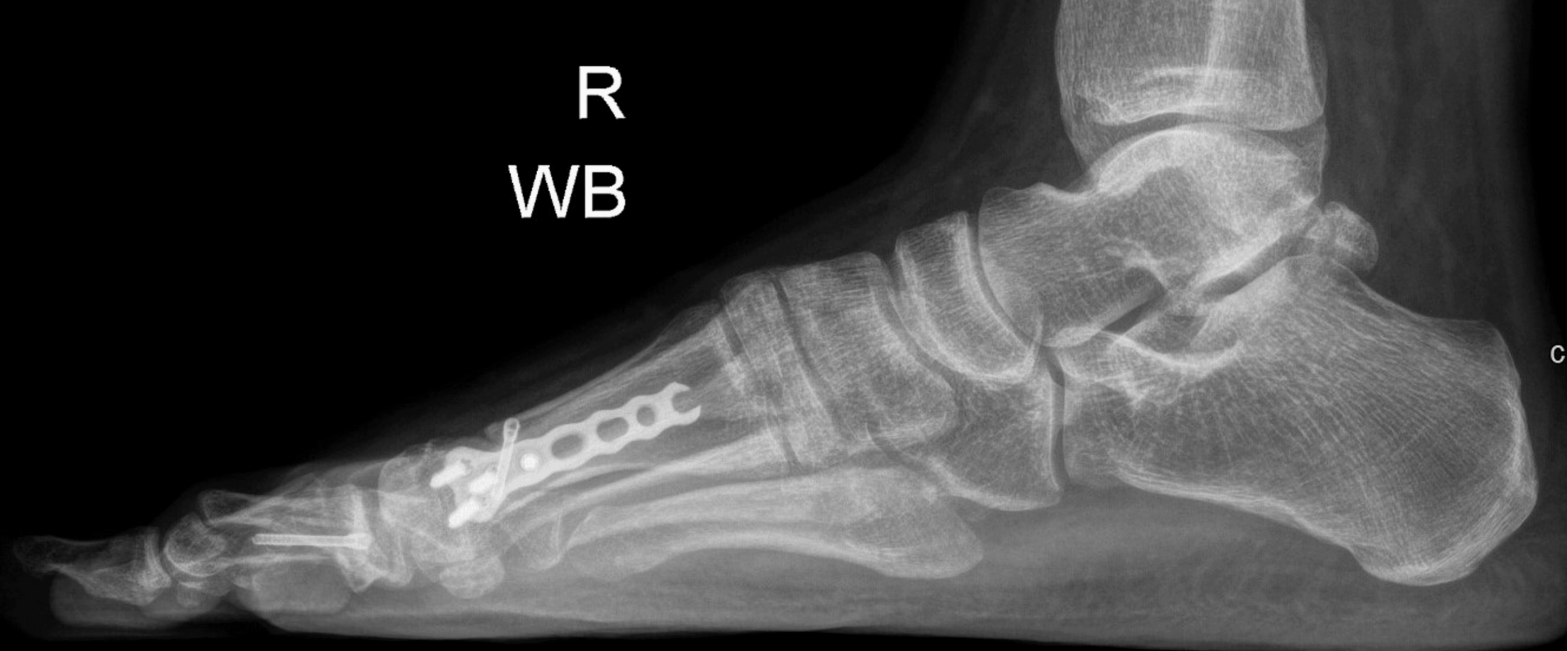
Post-operative weightbearing lateral foot radiograph showing the intramedullary plate inserted
